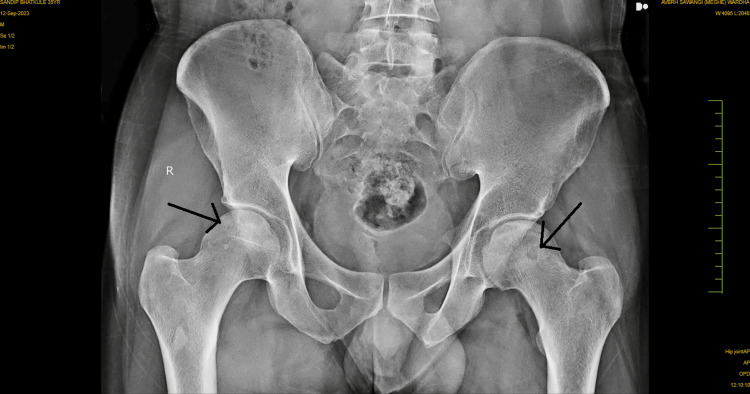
Anteroposterior radiograph of both hips showing cysts on both hips
